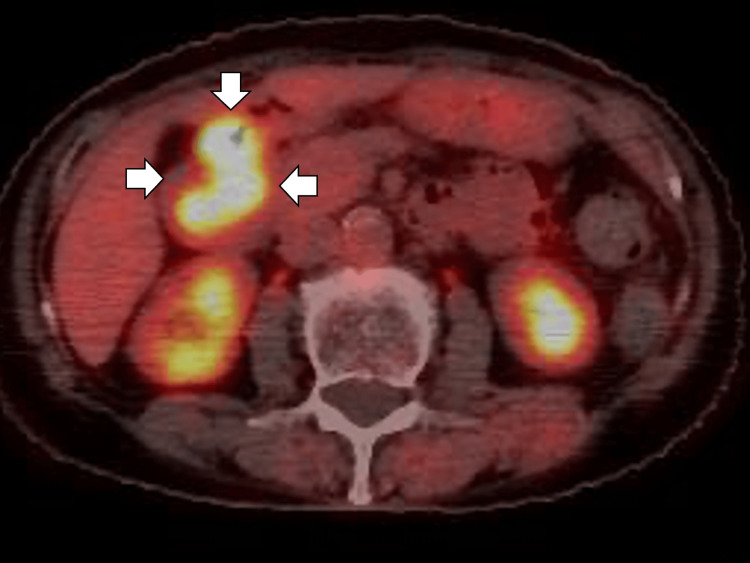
The positron emission tomography-computed tomography showing fluorodeoxyglucose uptake in the descending portion of the duodenum, with a maximum standardized uptake value of 15.8 and associated wall thickening, raising suspicion of a second primary malignancy (white arrows).
